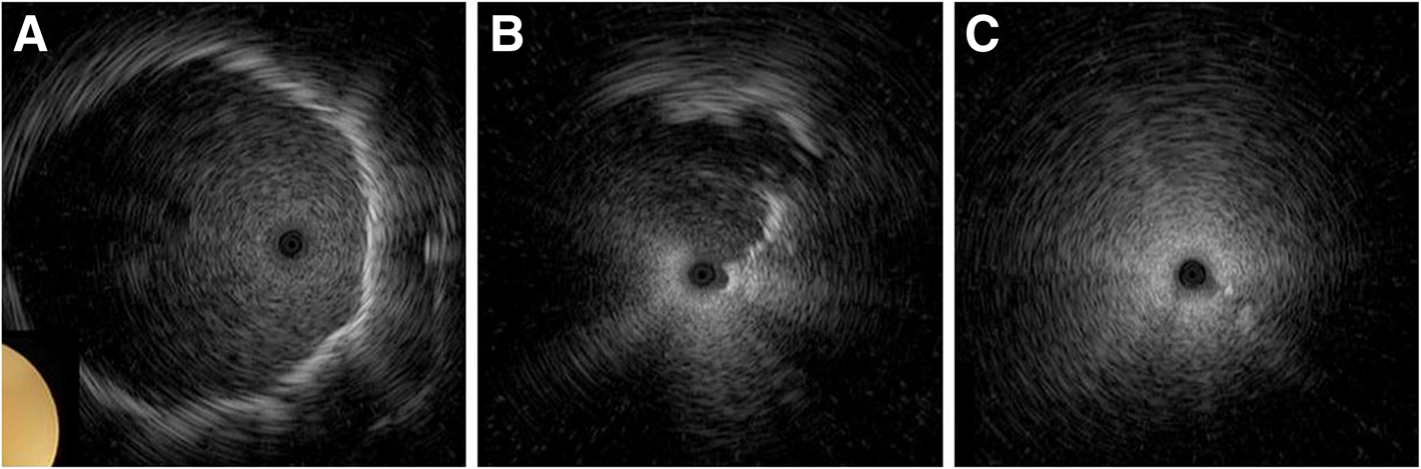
EBUS image according to the relationship between the peripheral lung lesion and the bronchus. The radial EBUS was positioned inside the peripheral lung lesion (a, within), in the bronchus adjacent to the lung lesion (b, adjacent to) and outside the lung lesion (c, outside). EBUS, endobronchial ultrasound
